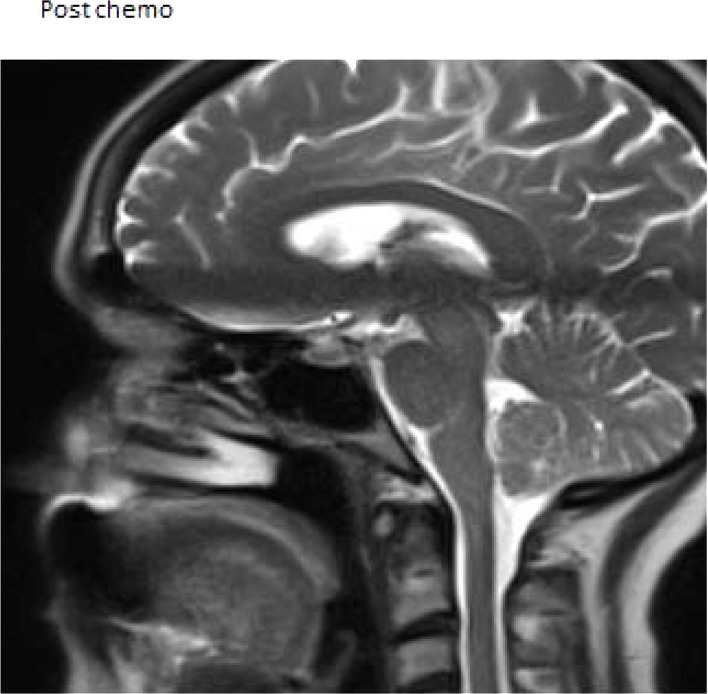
Case 3. Magnetic resonance image of the pituitary gland after chemotherapy showing an empty sella.
